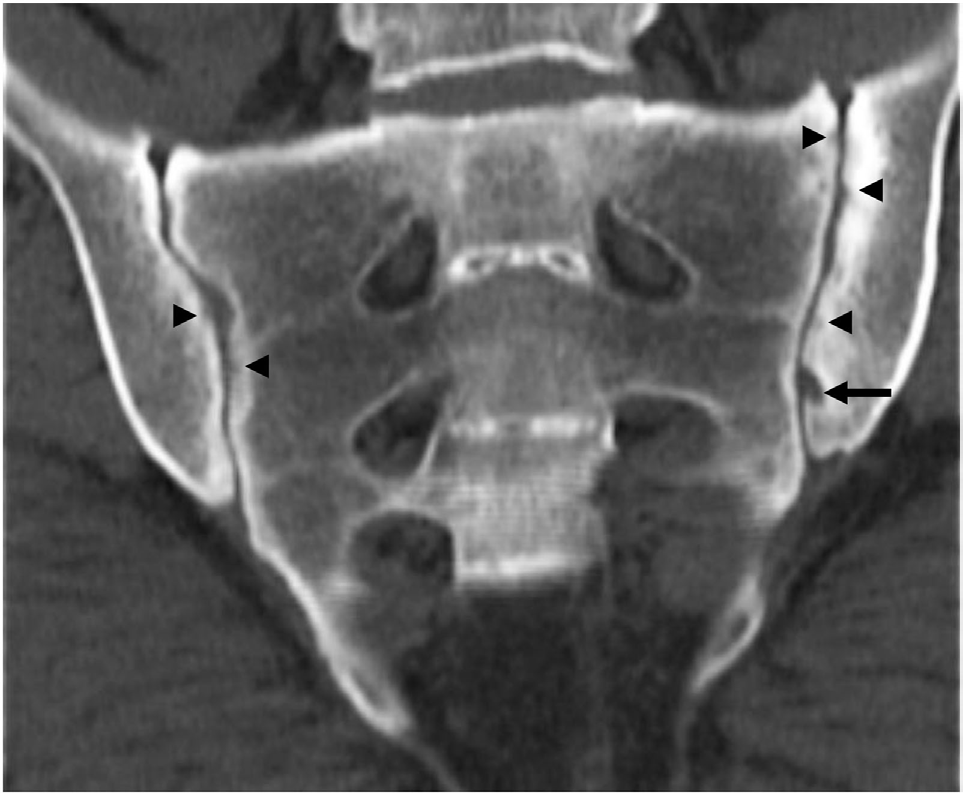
Computed tomography of the sacroiliac joints (same patient as depicted in Figures 1 and 2) demonstrating subchondral sclerosis predominantly of the left ilium, multiple bilateral small erosions (arrowheads), and a large erosion of the left ilium (arrow).
